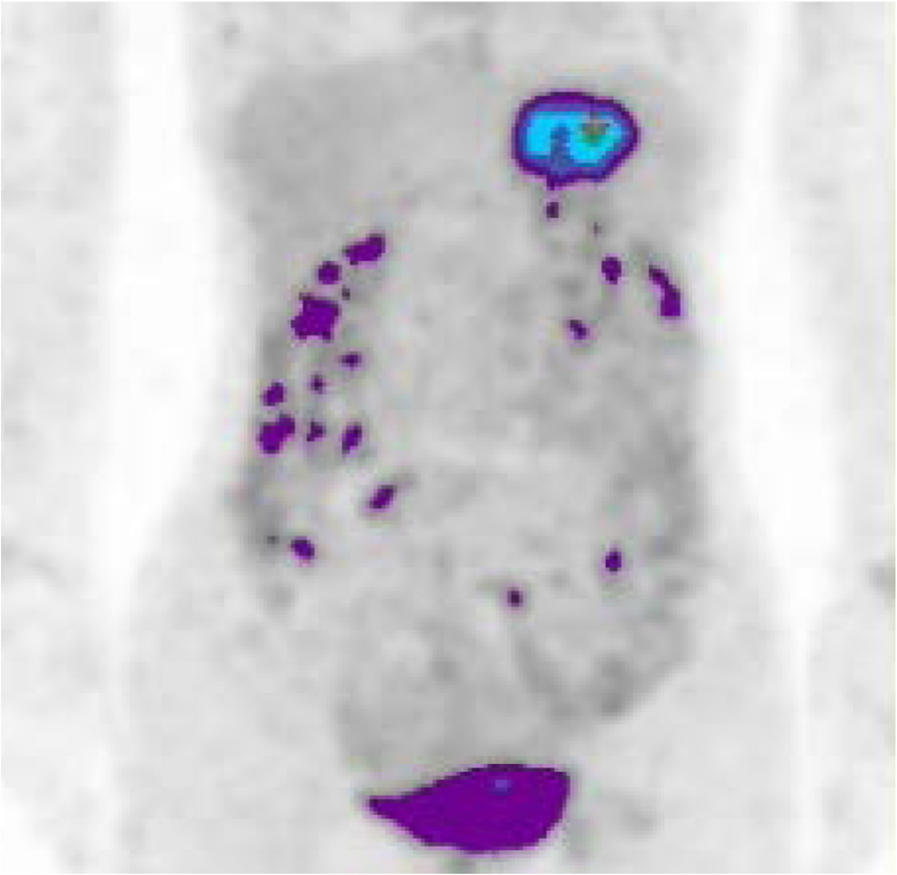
^18^F-FDG PET image showing avid FDG accumulation in the left hepatic mass (maximum standardized uptake value = 6.3)
